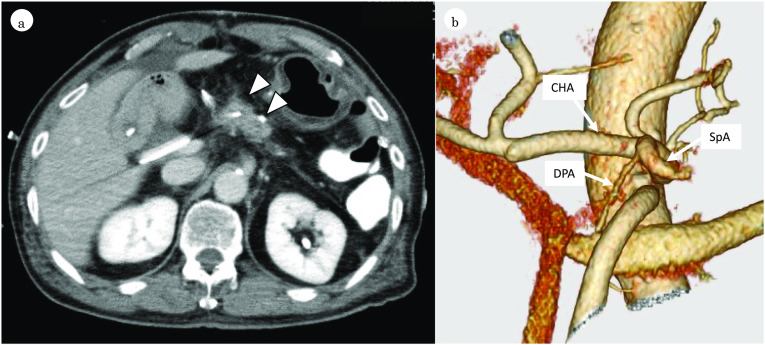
Postoperative CT revealed the enhancement of pancreatic remnant (arrowhead) (a). Postoperative 3D-CT showed that the DPA was successfully preserved (b). *DPA* dorsal pancreatic artery
